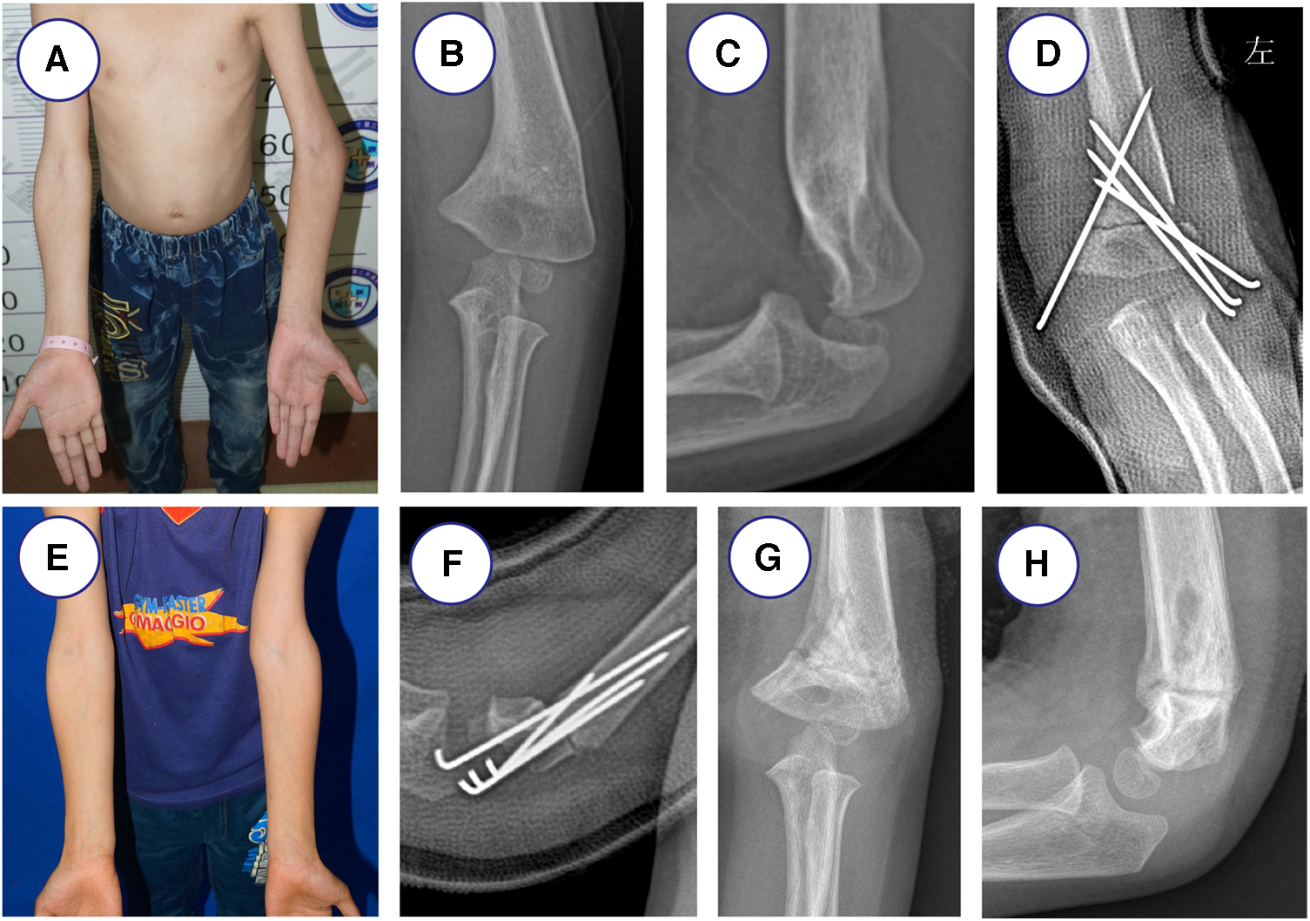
7-year-old boy with left cubitus varus treated with K-wires and cast fixation owing to left supracondylar humeral fracture. (A) Clinical photograph before surgery. (B,C) Radiographs showed left cubitus varus before surgery. (E) Clinical photograph after surgery at the last follow-up time. (D,F) Radiographs show K-wires and cast fixation after lateral closing-wedge osteotomy. (G,H) K-wires fixation were removed after 2 months.
